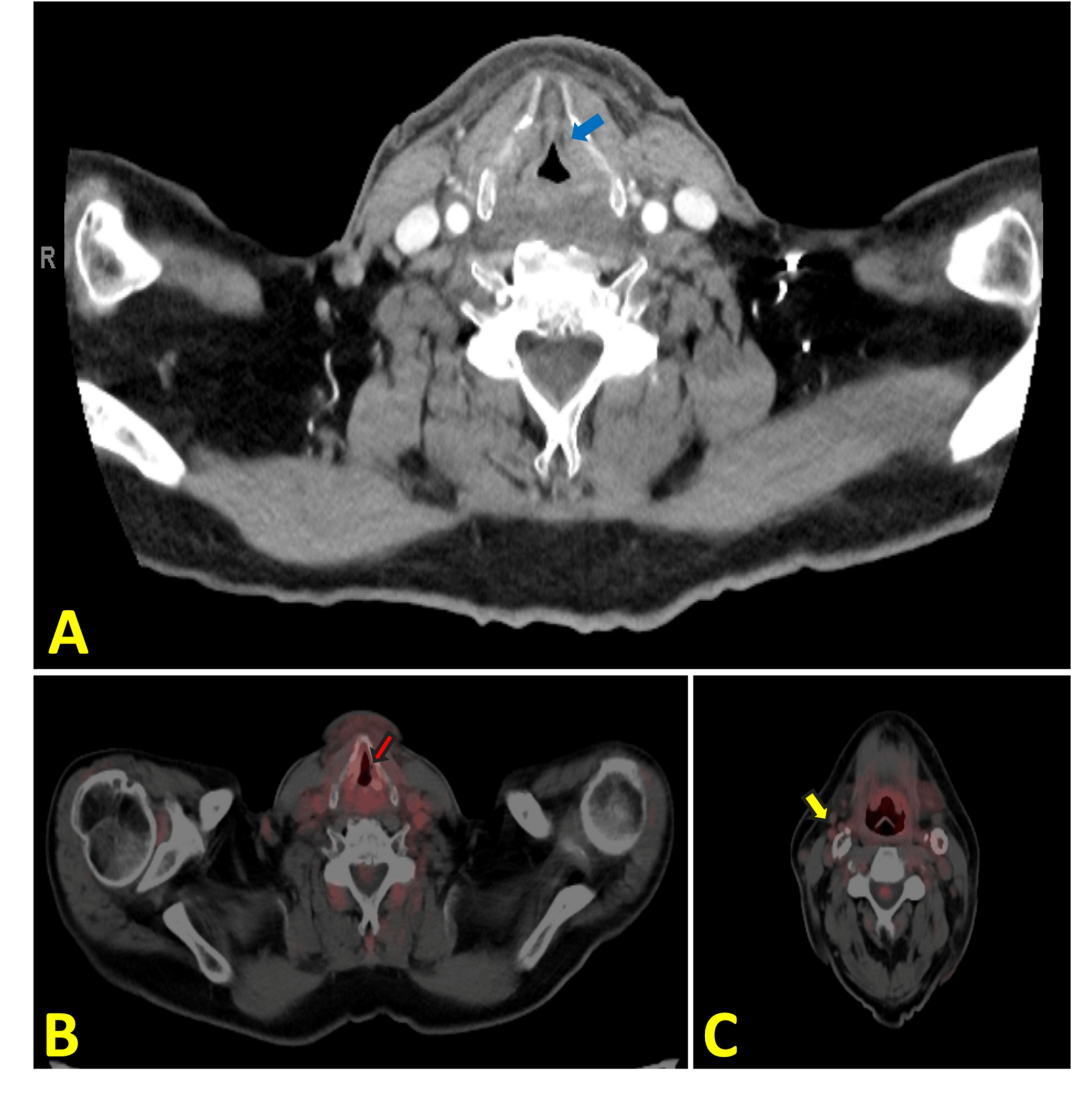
A: Post-treatment CT scan of laryngeal cancer with a blue arrow indicating a decrease in size of the left vocal cord lesion. B: Post-treatment PET-CT scan with a red arrow indicating improvement and resolution of FDG uptake in the laryngeal region. C: Post-treatment PET-CT scan with a yellow arrow indicating a decrease in size and resolution of FDG uptake in the right cervical node PET-CT: Positron Emission Tomography-Computed Tomography; FDG: Fludeoxyglucose
